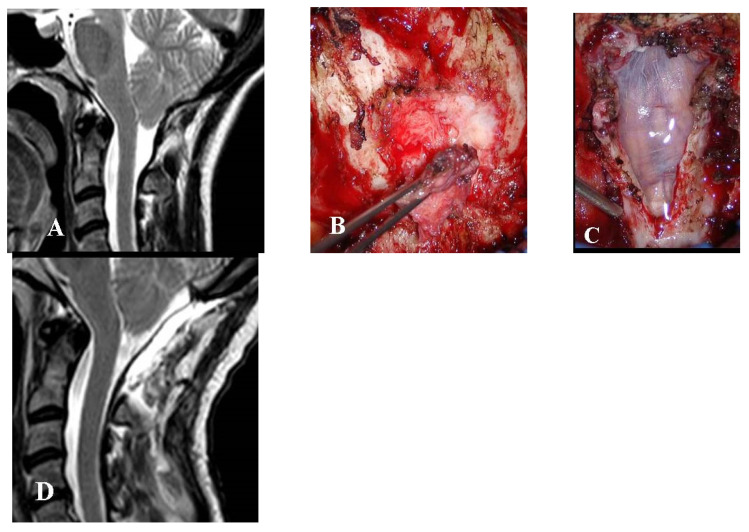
This 41-year-old woman had undergone bone decompression alone. Over the next 8 years, her headache recurred and progressively worsened. She also complained of dizziness and bouts of diplopia. (A) Preoperative MRI, T2-weight, sagittal view showing the bone decompression with scarce CSF film around the CM-1. (B) Intraoperative picture showing a thick and hard extradural fibrous scar that was maintaining the compression. (C) Intraoperative picture following dural opening with intact arachnoid. The dural sac was repaired using biologic graft. (D) Follow-up MRI, T2-weight, sagittal view obtained 6 months later showing the CM-1 was well bathed in CSF. Preoperative symptoms were almost completely resolved. The final CCOS score was 14.
